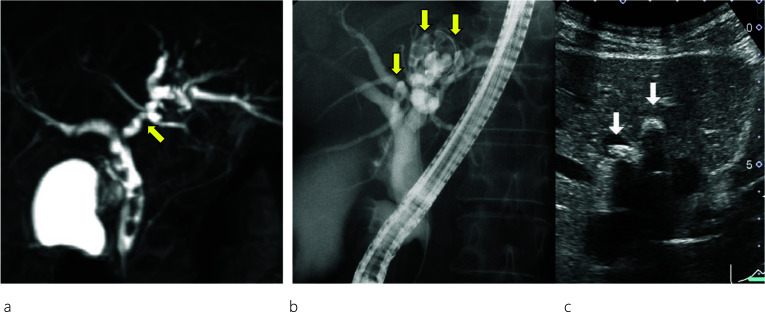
Image findings. Magnetic resonance cholangiopancreatography (MRCP) (a) shows common bile duct (CBD) stones and stenosis at the confluence of B2 and B3. Endoscopic retrograde cholangiopancreatography (ERCP) (b) reveals intrahepatic bile duct (IHBD) stones in B2 and B4 (yellow arrows). Abdominal ultrasound scan (c) shows IHBD stones in B2 and B4 (white arrows)
